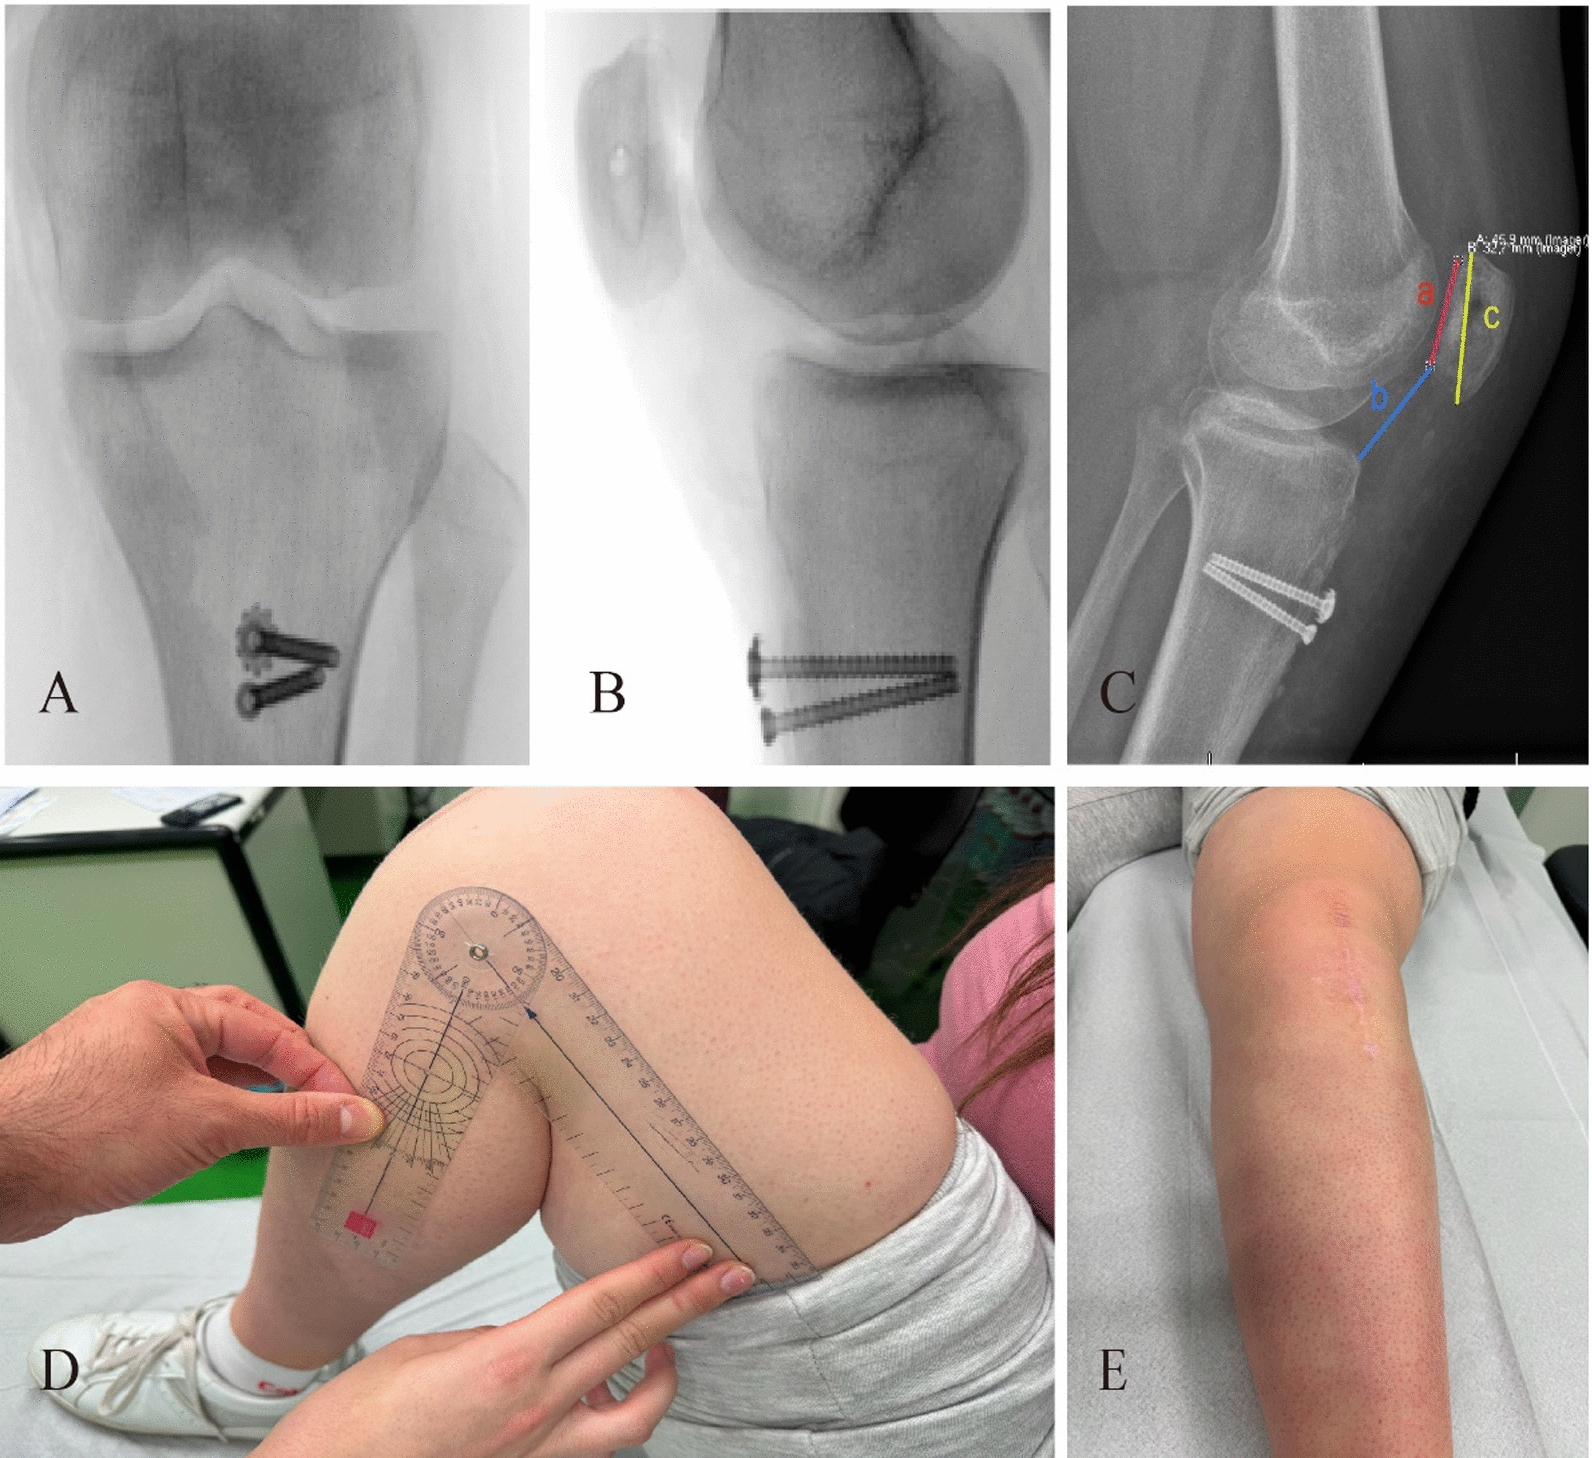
A The preoperative lateral radiograph of the left knee with a patellar Caton–Deschamps index (b/a) of 1.7 and Insall–Salvati index (d/c) of 0.8. B The preoperative lateral radiograph of the left knee with a patellar modified Insall–Salvati index (f/e) of 2.3 and Blackburne–Peel index of 1.6. C Preoperative anteroposterior view radiograph of the bilateral knee showed that the height of the patella in the left knee was 15 mm higher than that in the right knee (indicated by the black lines). D Preoperative sagittal plane magnetic resonance imaging revealed a morphology ratio (i/j) of 2. E The preoperative sagittal plane of the computed tomography scan
